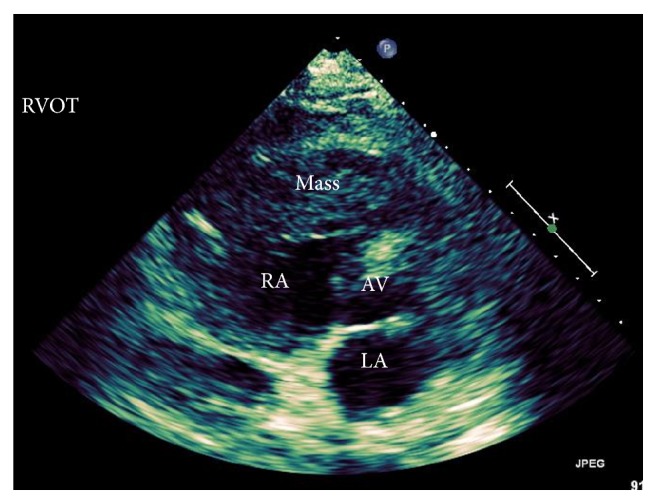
Technically difficult, parasternal short axis view at the aortic valve level showing a large heterogeneous mass occupying a majority of the RVOT.
